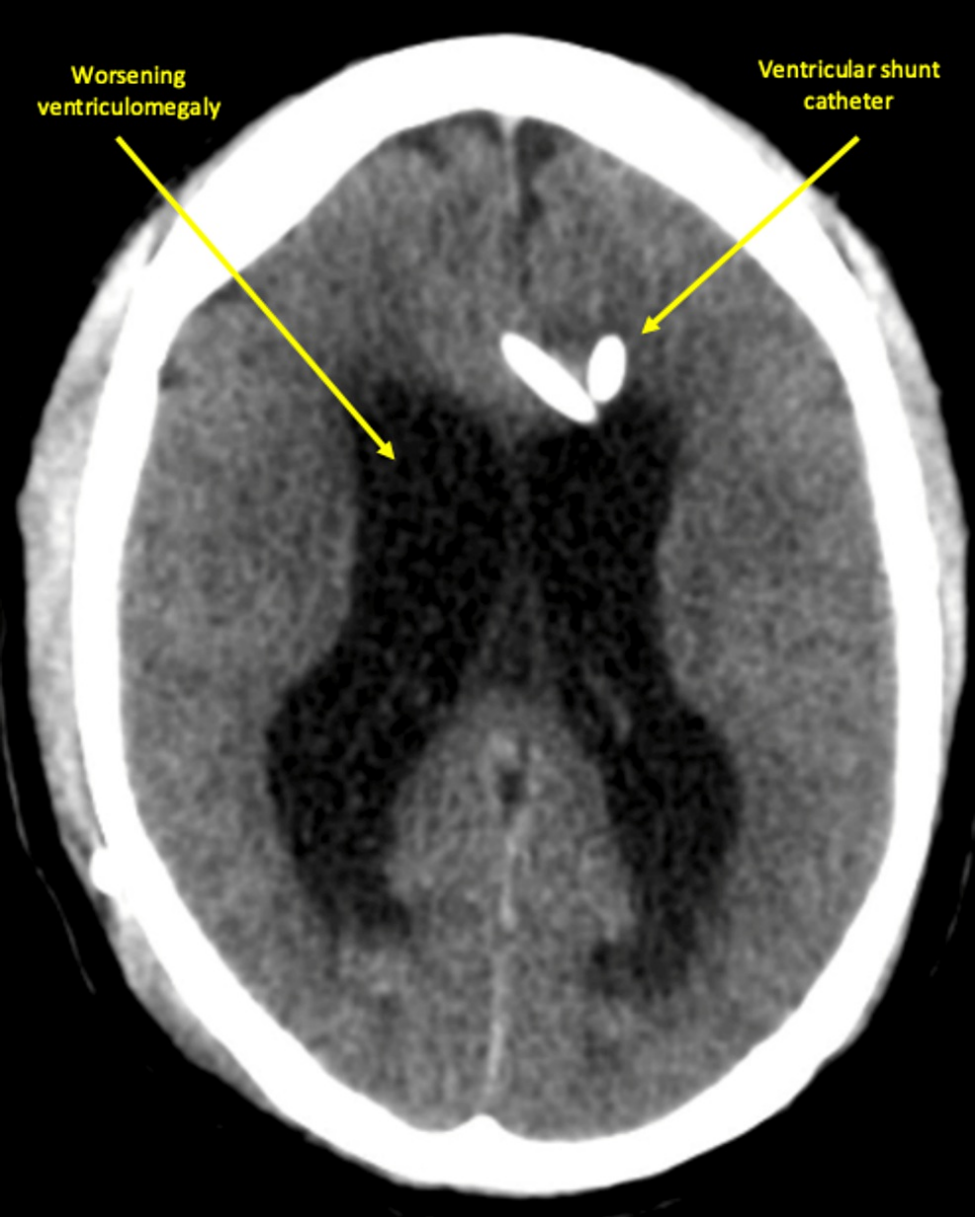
CT head without contrast on the first day of readmission displayed ventricular shunt catheter with new intraparenchymal hemorrhage at the right frontal lobe and extra-axial hemorrhage anterior to the left frontal lobe. In addition, worsening ventriculomegaly concerning for worsening hydrocephalus was also seen.
